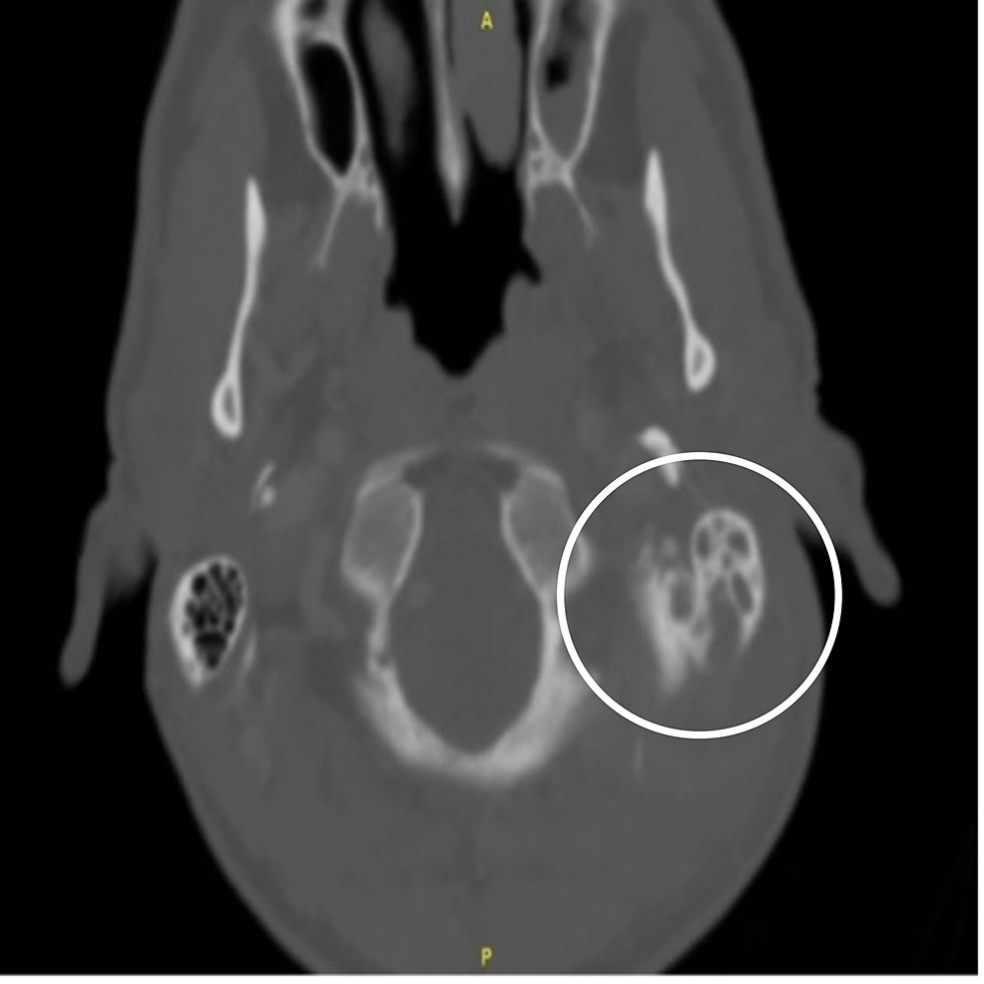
Axial CT at the level of the mastoid showing posterior defect of the left mastoid cortex with complete mastoid opacification.
